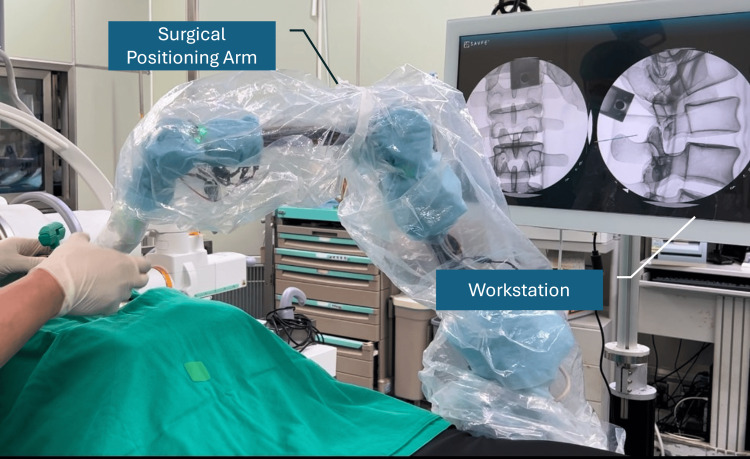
Overview of the ArmSure fluoroscopic positioning system, including the passive surgical positioning arm and workstation with integrated display and processing unit. The system is designed for X-ray-guided spinal navigation without optical tracking
